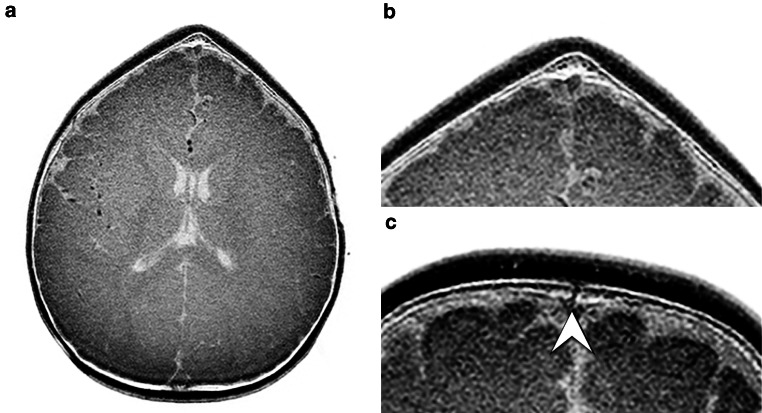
Inverted axial reformation of the 3D T1-weighted gradient echo sequence of the skull of a 5-month-old male patient with metopic synostosis and trigonocephalus (a), showing the fused metopic suture of this patient in an axial plane (b). Inverted axial reformation of the 3D T1-gradient echo sequence of the skull of a 6-month-old male patient with a patent metopic suture (c), indicated by the arrowhead. It should be noted that the lamina interna, diploe and lamina externa can clearly be differentiated from each other on these images
